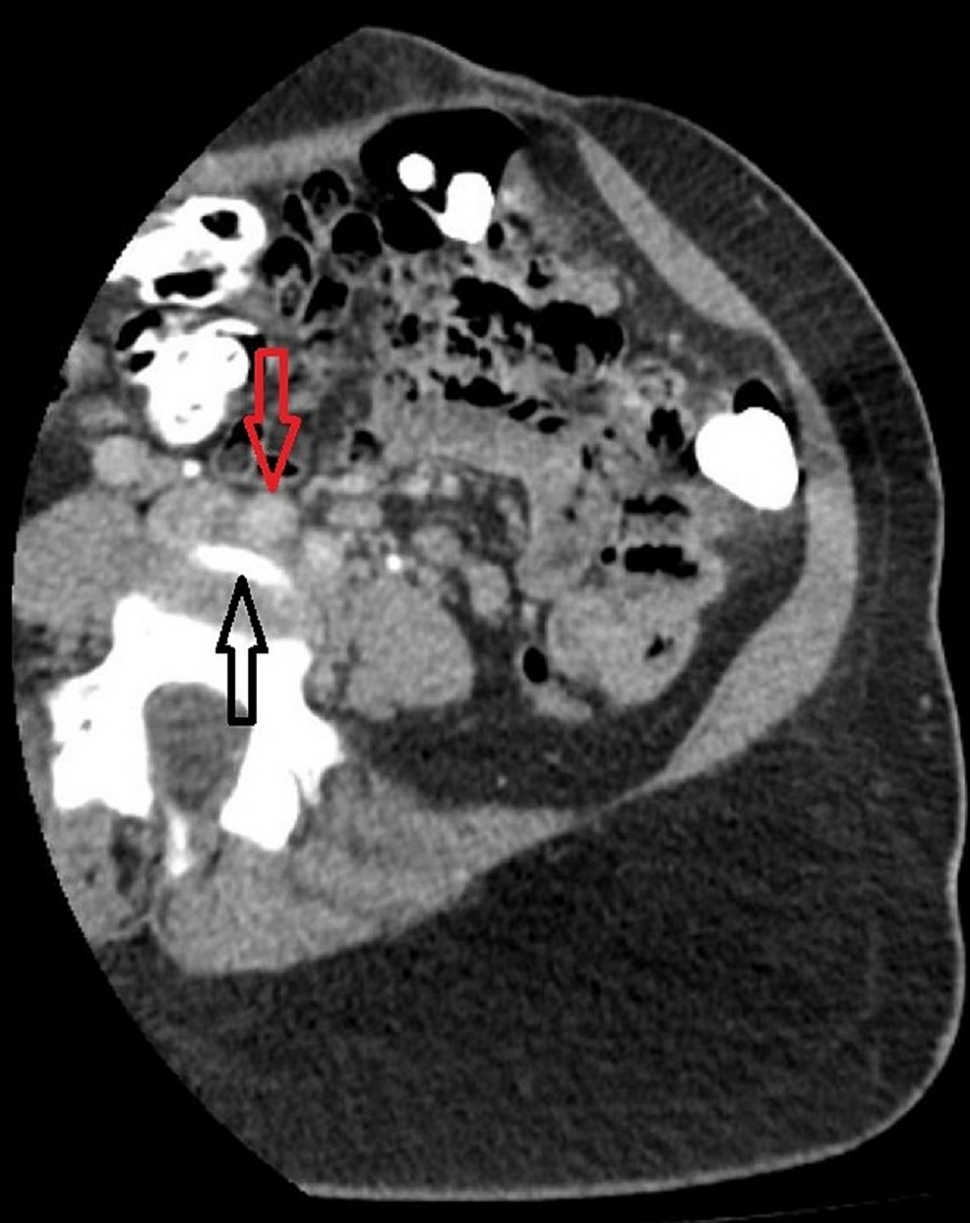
Transverse section of CT pelvis showing compression of the left common iliac vein (black arrow) by the right common Iliac artery (red arrow) in the pelvis. CT: computed tomography
